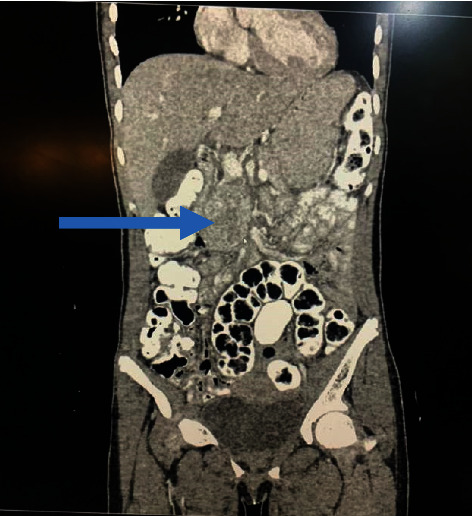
Contrast-enhanced coronal reformatted computed tomography of the abdomen. The mass (depicted by the blue arrow) is slightly to the right of the midline.
